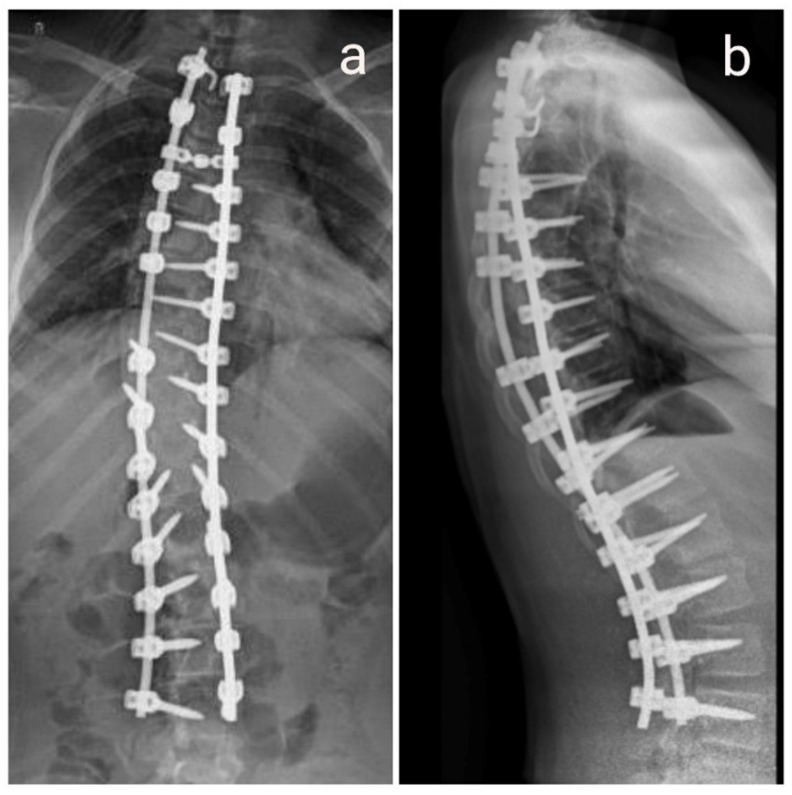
Postoperative X-ray. (a) AP X-ray image. (b) L X-ray image.
